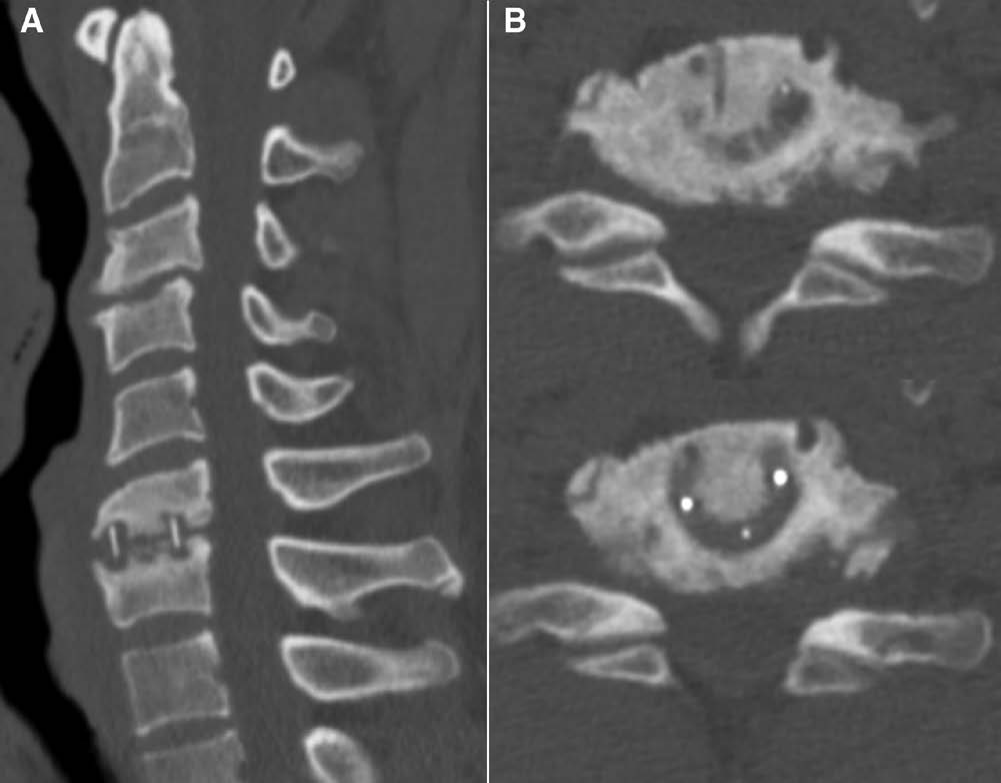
(A) Post-operative computed tomography (CT) image obtained 10 months after surgery showing subsidence at the C6–7 level. (B) Axial CT image at the C6–7 level showing that right-side foraminal stenosis occurred alongside subsidence when uncoforaminotomy was insufficiently performed.
